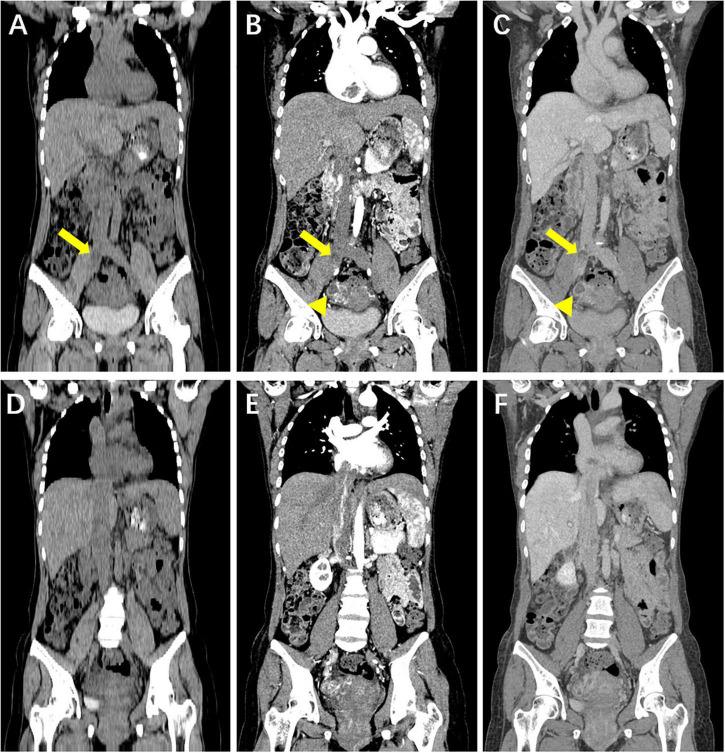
Coronal reformatted computed tomography (CT) image of the chest, abdomen and pelvis. (A–F) Hypoattenuating filling defect located within the right iliac veins across the inferior vena cava (IVC) into the right atrium. Contrast-enhanced CT images show that intravenous lesions demonstrated mainly moderate progressive enhancement, with localized non-enhancing necrotic areas (arrow). A circular enhanced thick-walled cystic lesion at the right of the uterine body is incidentally noted (arrowhead). (A,D) Unenhanced phase image. (B,E) Arterial phase of contrast enhancement image. (C,F) Portal phase of contrast enhancement image.
